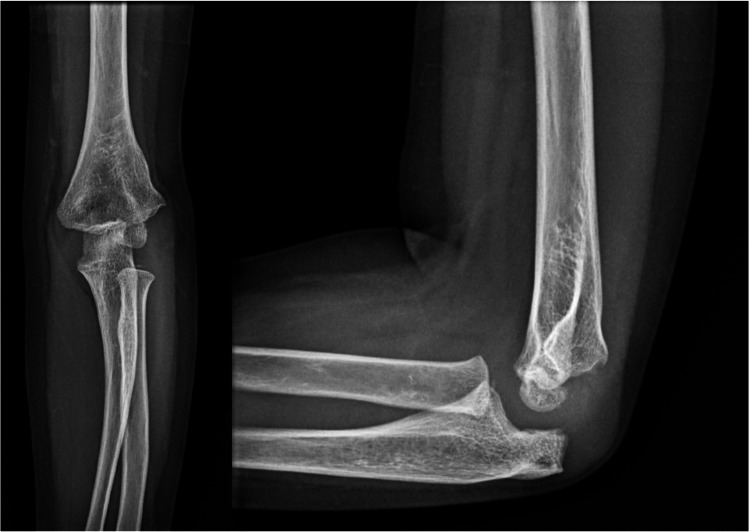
Six-week postoperative follow-up (A) Anteroposterior and (B) lateral radiographs of the elbow, demonstrating complete healing of both fractures with no evidence of malunion
